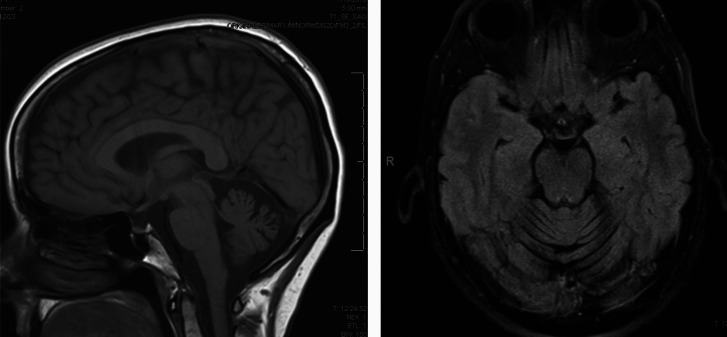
Sagittal T1 MRI and axial FLAIR MRI brain sequences show cerebellar atrophy in a patient with functional gait disorder without overt cerebellar symptoms
